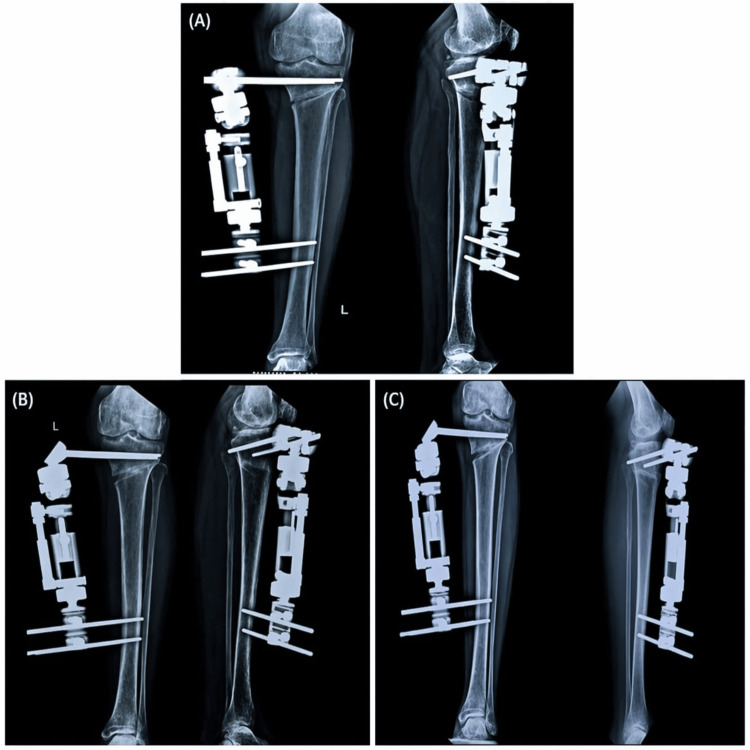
Follow-up radiographs. (A) at one month; (B) at three months; (C) at six months
